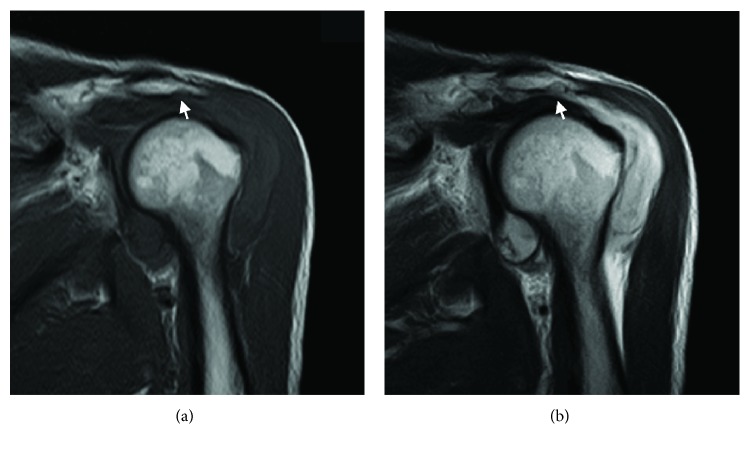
Oblique coronal magnetic resonance images of the left shoulder. (a) T1-weighted images. (b) T2-weighted images. An extensive hematoma was seen in the glenohumeral joint and the subacromial and subdeltoid bursas, which was shown as low signal intensity in T1-weighted images and high signal intensity in T2-weighted images. Bony erosion on the undersurface of the acromion was detected (white arrows).
